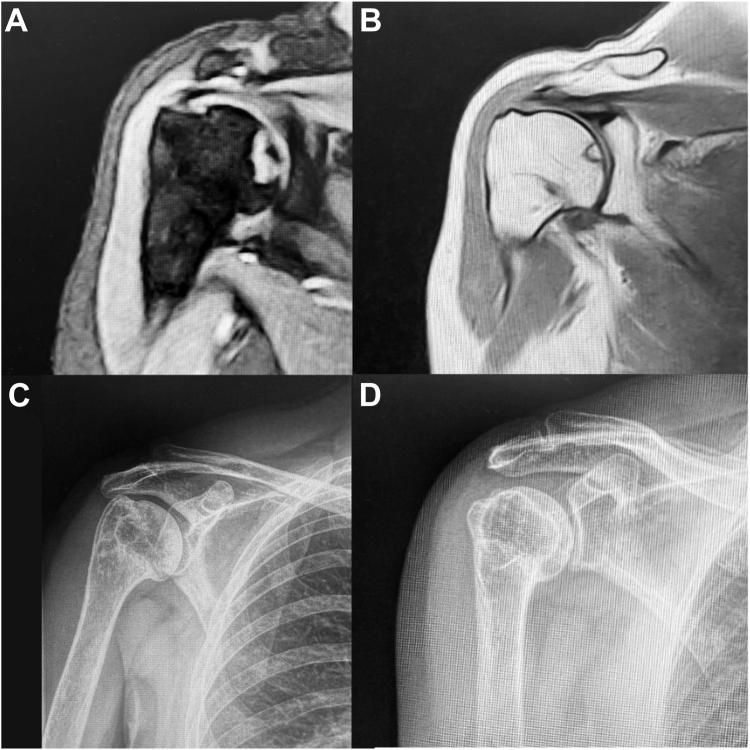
Right shoulder. Final MRI (A and B) and X-Rays (C and D) 8.5 years FU. A focal area of avascular necrosis without collapse is present. *FU*, follow-up; *MRI*, magnetic resonance imaging.
